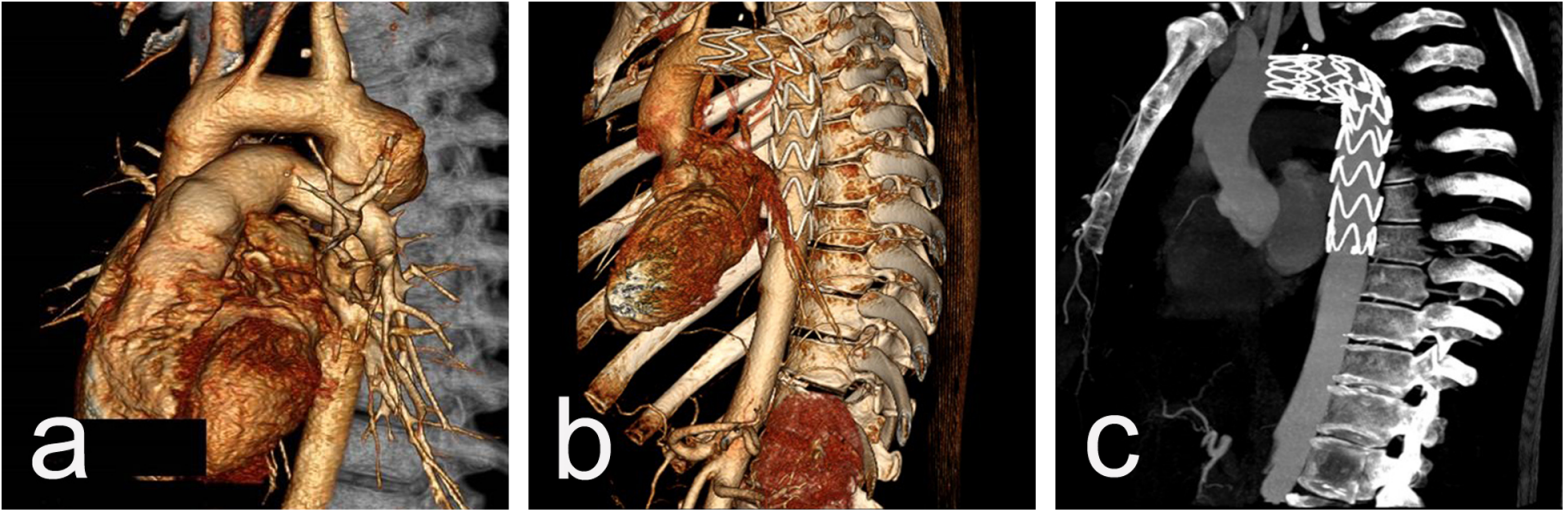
CT scan imaging before (a) and after (b and c) stent-graft implantation of 26y/o male presenting acutely with contained rupture of an aneurysm 20 years after initial CoA repair. The aortic aneurysm is located close to the origin of LSA (a) and the implanted graft excluded the aneurysmal sac but fully covered the LSA (b) with an excellent blood supply through the left vertebral artery from control CTAs (c).
